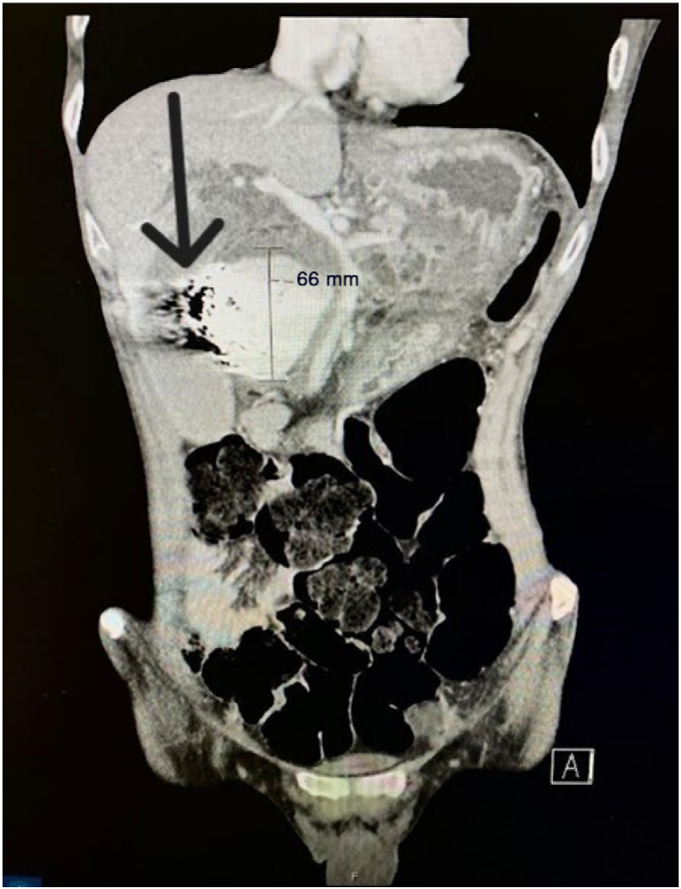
Coronal contrast-enhanced computed tomography in the arterial phase, pre-procedure, demonstrating a large visceral pseudoaneurysm in the right upper quadrant (brackets with measurements); and an artifact from the endovascular coils within the lesion from prior embolization (arrow).
